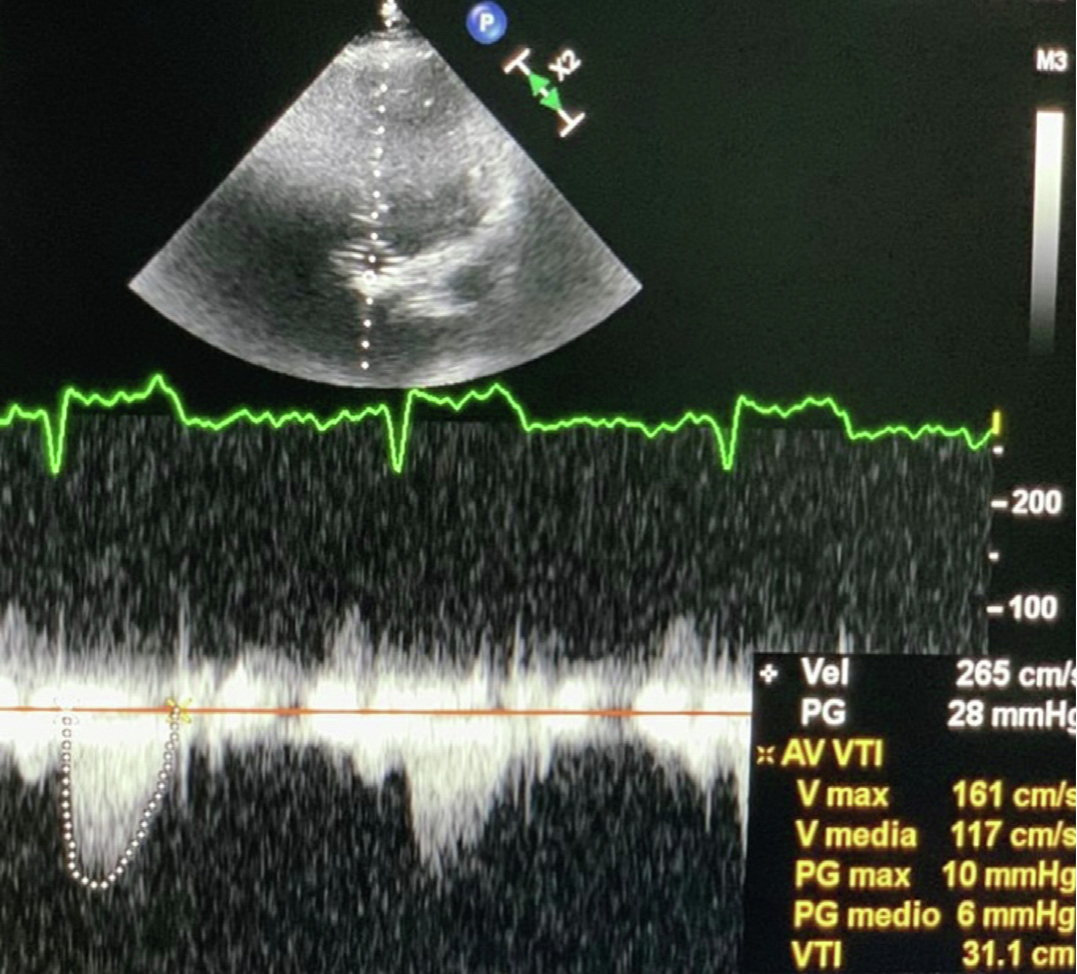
Continuous Doppler Through the Inner Aortic Bioprosthesis at 1-Year Follow-up Showing Normal Mean Gradient PG = pressure gradient; other abbreviations as in Figure 1.
